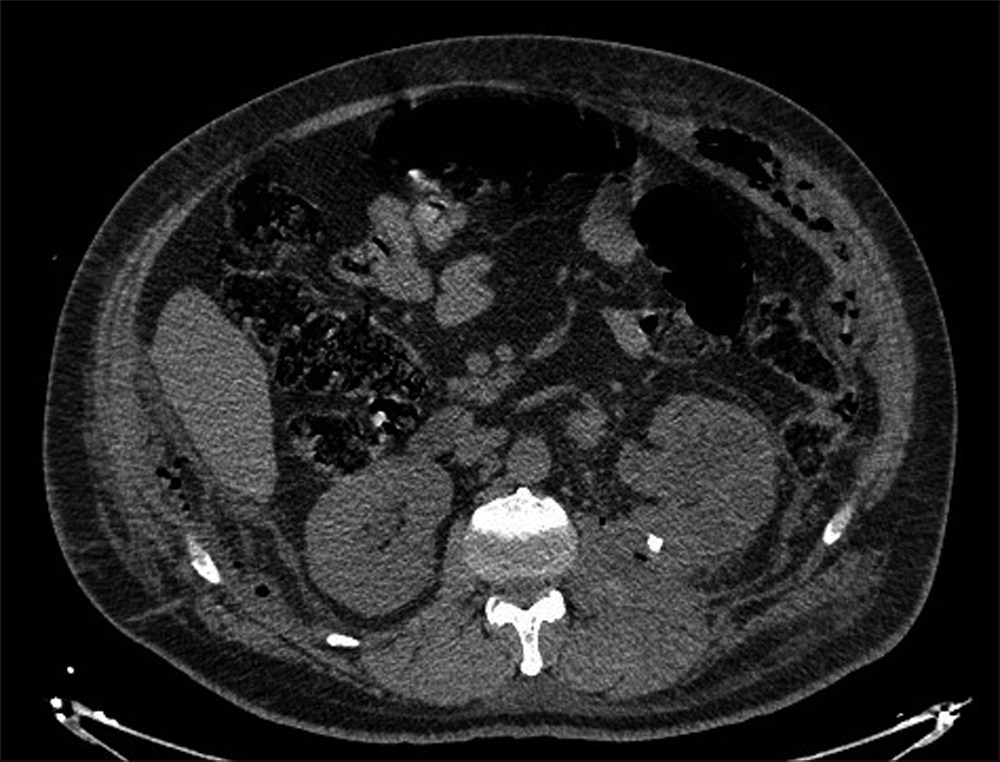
Computed tomography showed left pyonephrosis with middle caliceal stones and perinephric phat infiltration
